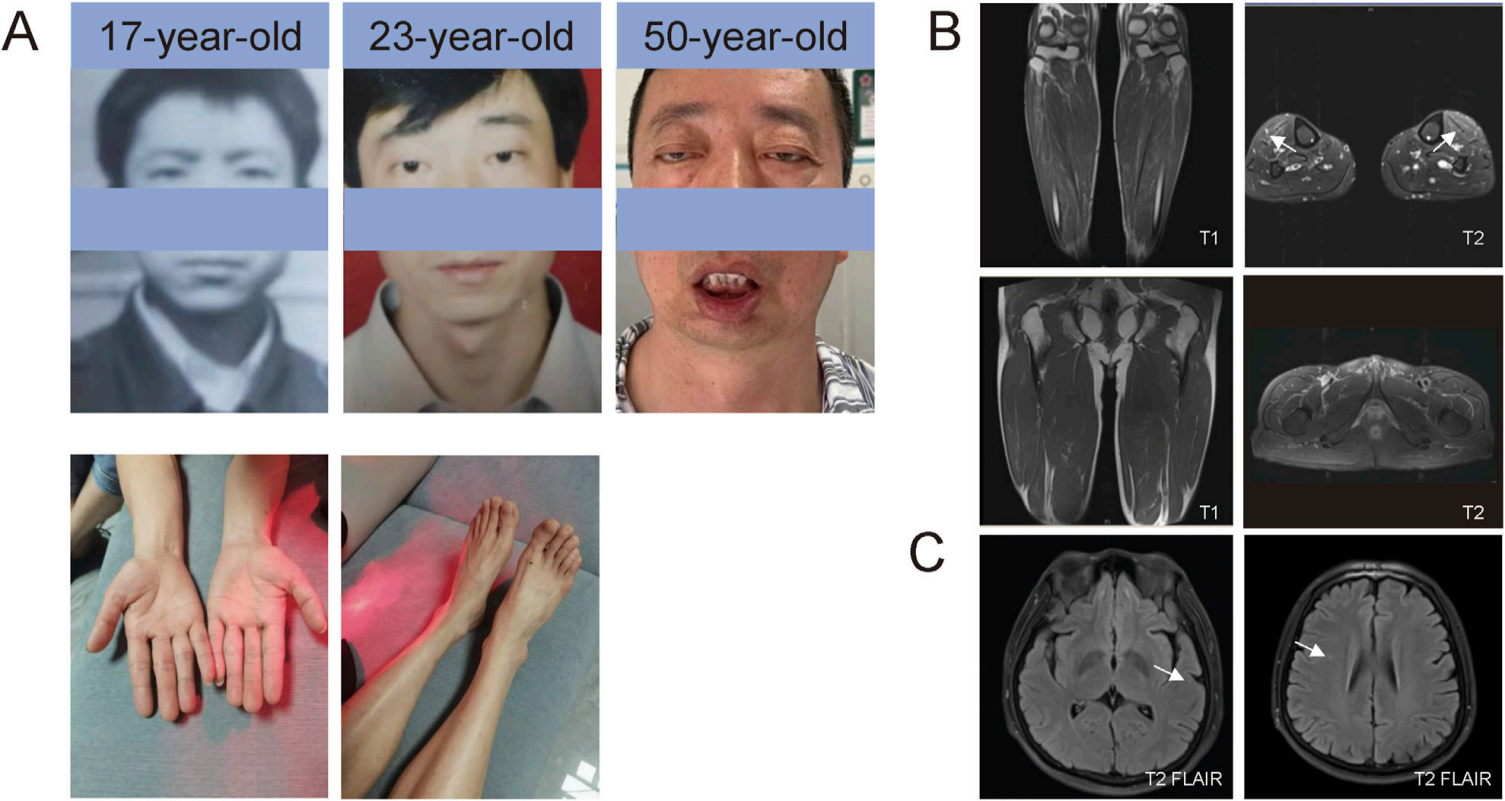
Photos of patients and MRI. (A) Facial photos at different ages and of the distal limbs. (B) T1- and T2-weighted MRI sequences of the legs. Muscle MRI showed T2 hyperintensities, suggesting edema of the lower legs (tibialis anterior). (C) T2/FLAIR sequences of the brain MRI. Brain MRI revealed T2/FLAIR hyperintensities, suggesting mild white matter demyelination.
